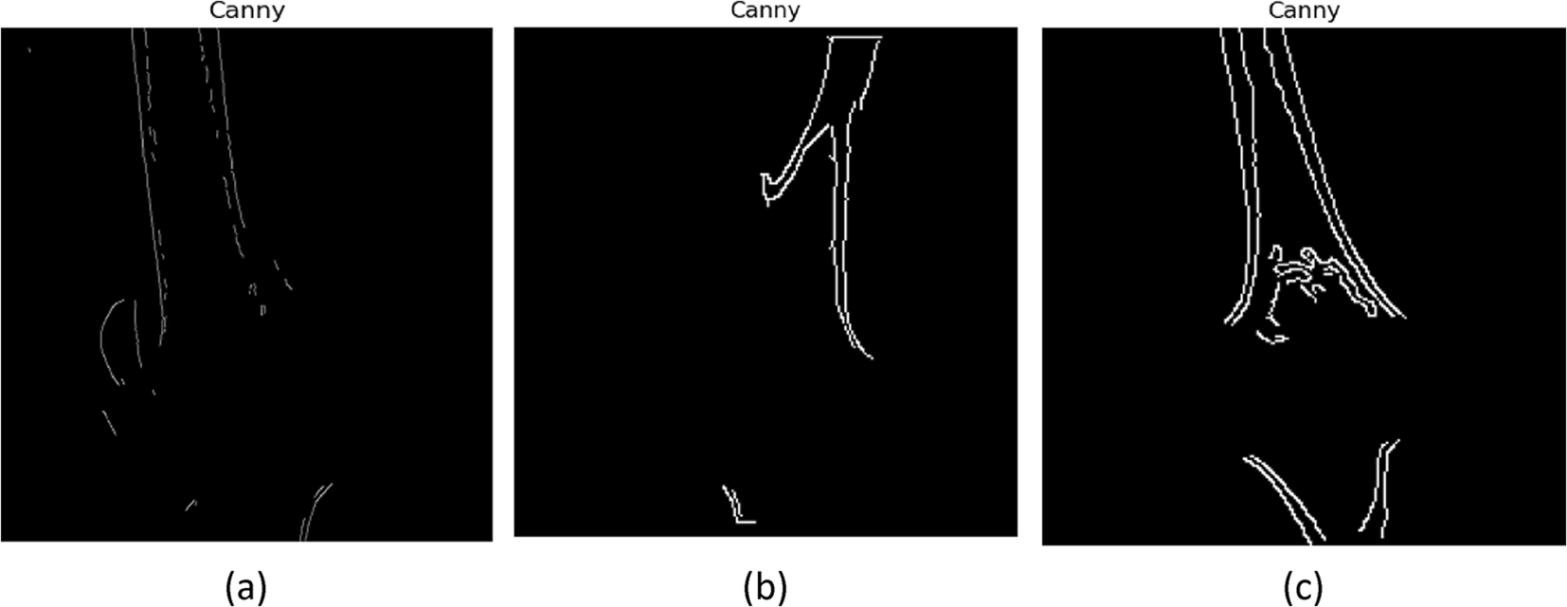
Canny edge detection: (a) lateral CT of parosteal osteosarcoma, (b) coronal CT of osteochondroma, and (c) lateral CT of enchondroma.
